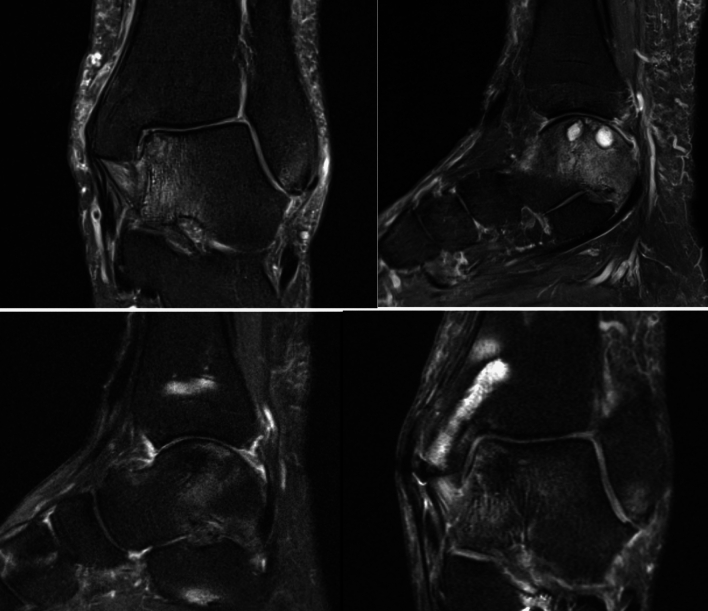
Coronary and sagittal slices of t2 sequenced MRI preoperatively and postoperatively after treatment of an osteochondral defect with subchondral cysts in the medial talus with autologous cancellous bone from the proximal tibia and minced cartilage along with fibrin fixation after osteotomy of the distal tibia
